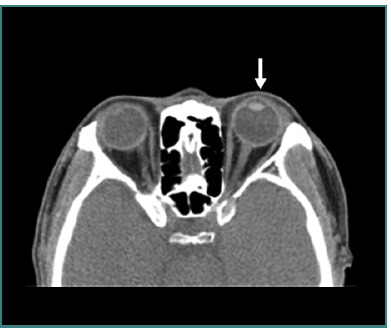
Axial computed tomography scan without contrast of left open globe (false negative). The arrow represents the missed shallow anterior chamber depth (ACD) of the left eye due to lens misalignment during the scan. Image reproduced with permission from the American Roentgen Ray Society: Yuan WH, Hsu HC, Lin YY, Liao SW. CT of globe rupture: analysis and frequency of findings. AJR Am J Roentgenol. 2014;202(5):4-5. ^©^ 2014 American Roentgen Ray Society.
